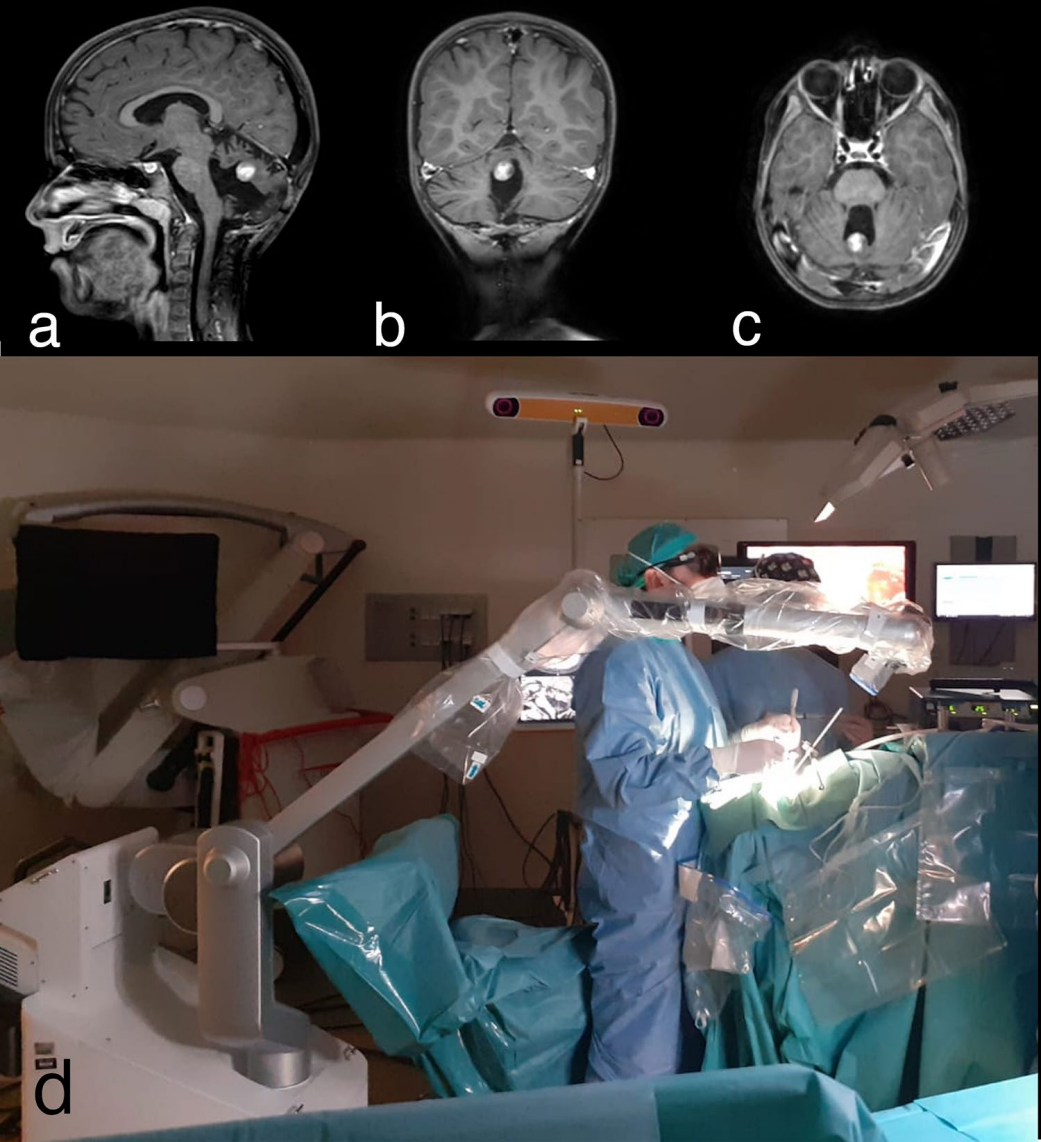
Preoperative T1 post-contrast magnetic resonance images of a recurrent pilocytic astrocytoma in the upper vermis (a in sagittal plane, b in coronal plane, and c in axial plane) and position of leading surgeon and exoscope in the operating room (d)
